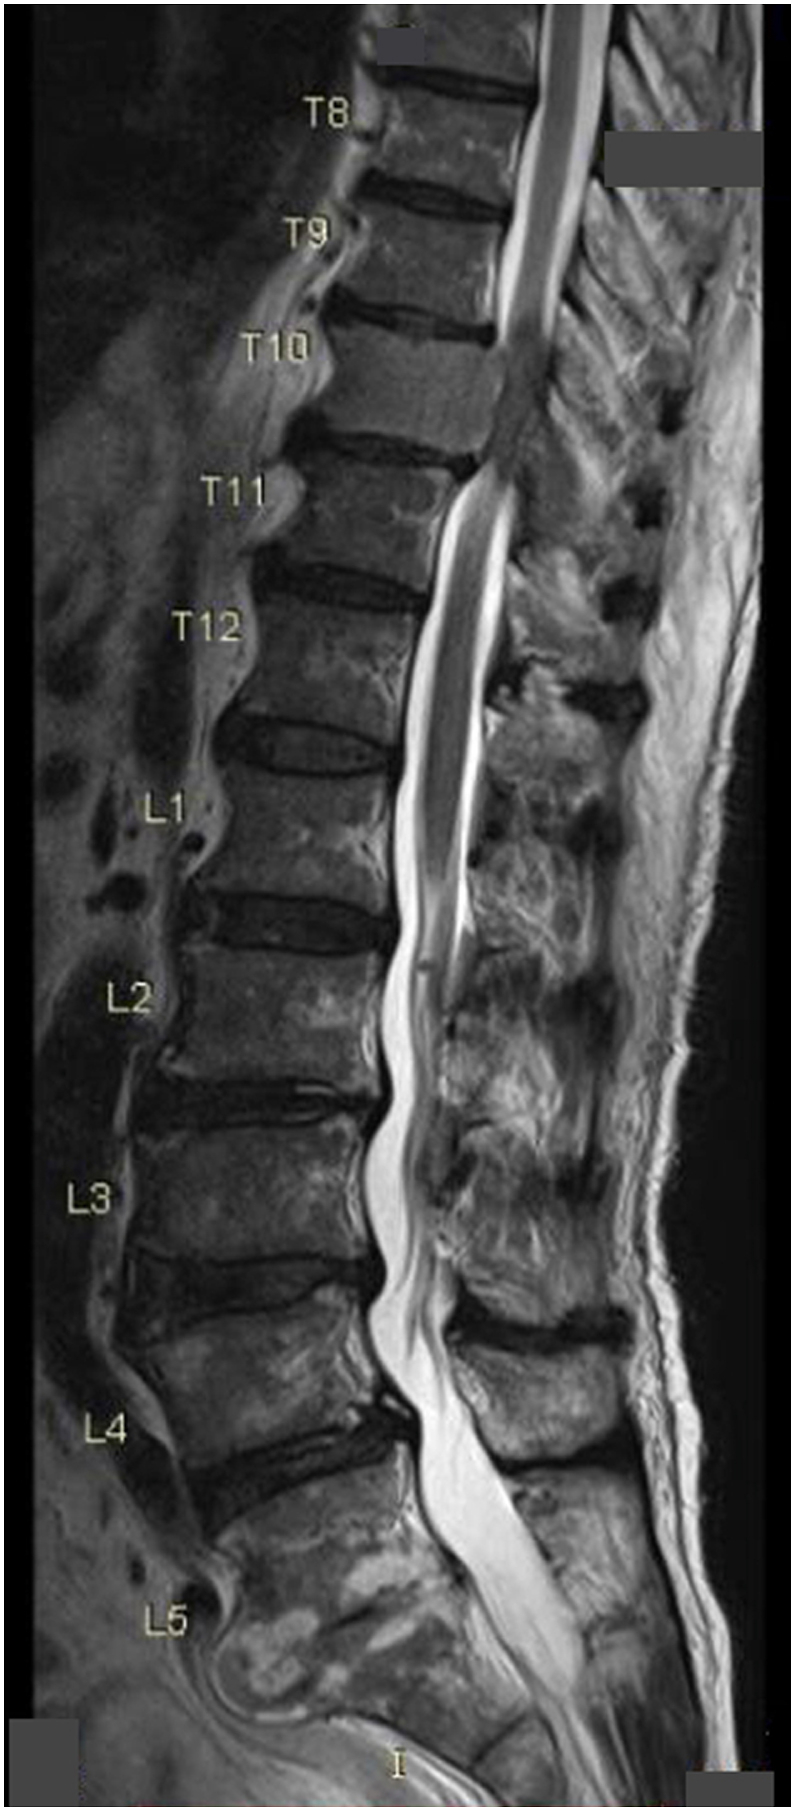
Sagittal view of the spine MRI showing severe spinal cord stenosis at T10 level.
